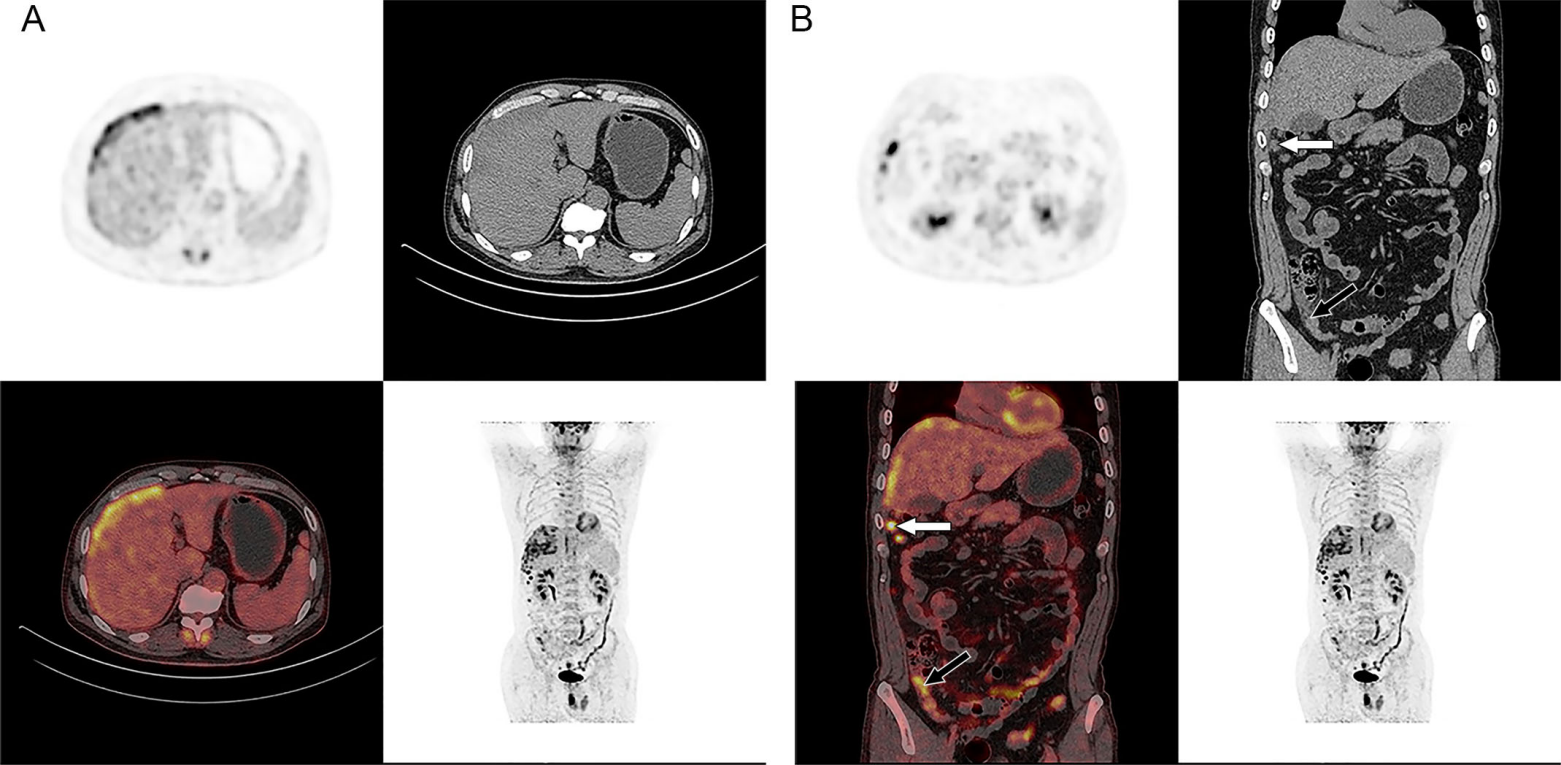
18F-FDG PET/CT images of the whole body. Axial PET/CT images of the abdomen showed abnormally elevated FDG activity in the multiple ill-defined low-attenuation lesions of the right subdiaphragm and right anterior and left medial lobe of the liver (SUVmax of 11.2 and SUVmax of 12.6 in late phase) (A). Coronal PET/CT images of the whole body showed abnormal uptake in the right peritoneum with irregular nodular thickness (black arrow) (SUVmax of 7.7 and SUVmax of 9.3 in late phase) and multiple high activity nodules in the right abdominal cavity (white arrow) (the largest one is SUVmax of 11.9 and SUVmax of 11.6 in late phase) (B).
